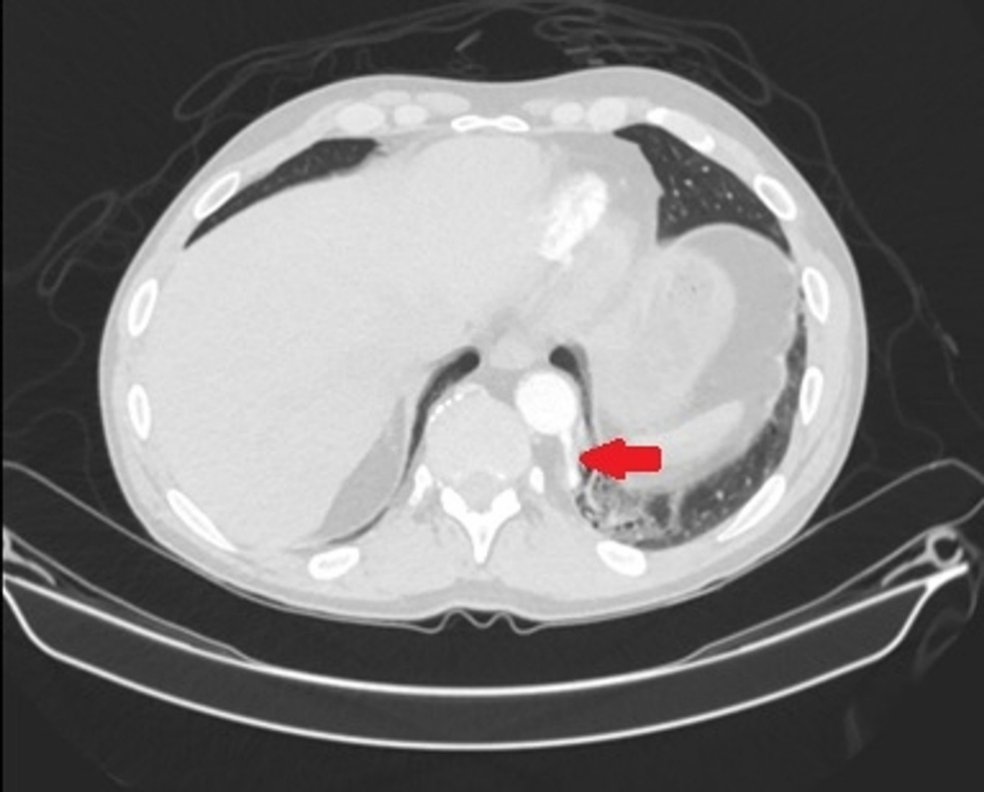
Axial CT scan of the abdomen with contrast demonstrating feeding artery arising from the aorta into intralobar sequestration. There is a subtle appreciation of more than one feeding vessel.
